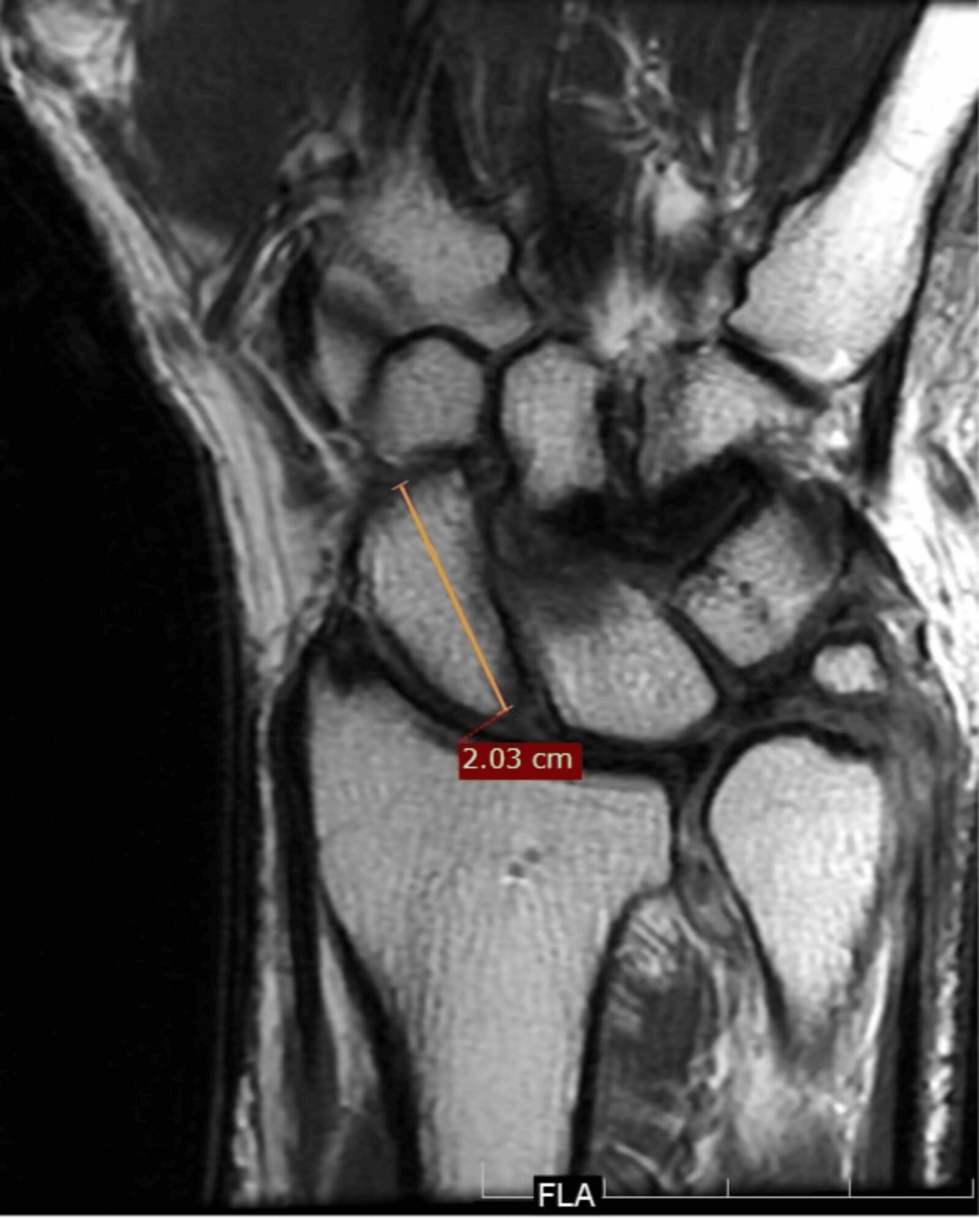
Technique showing the measurement of the scaphoid length on coronal T1-weighted MRI sequences.
